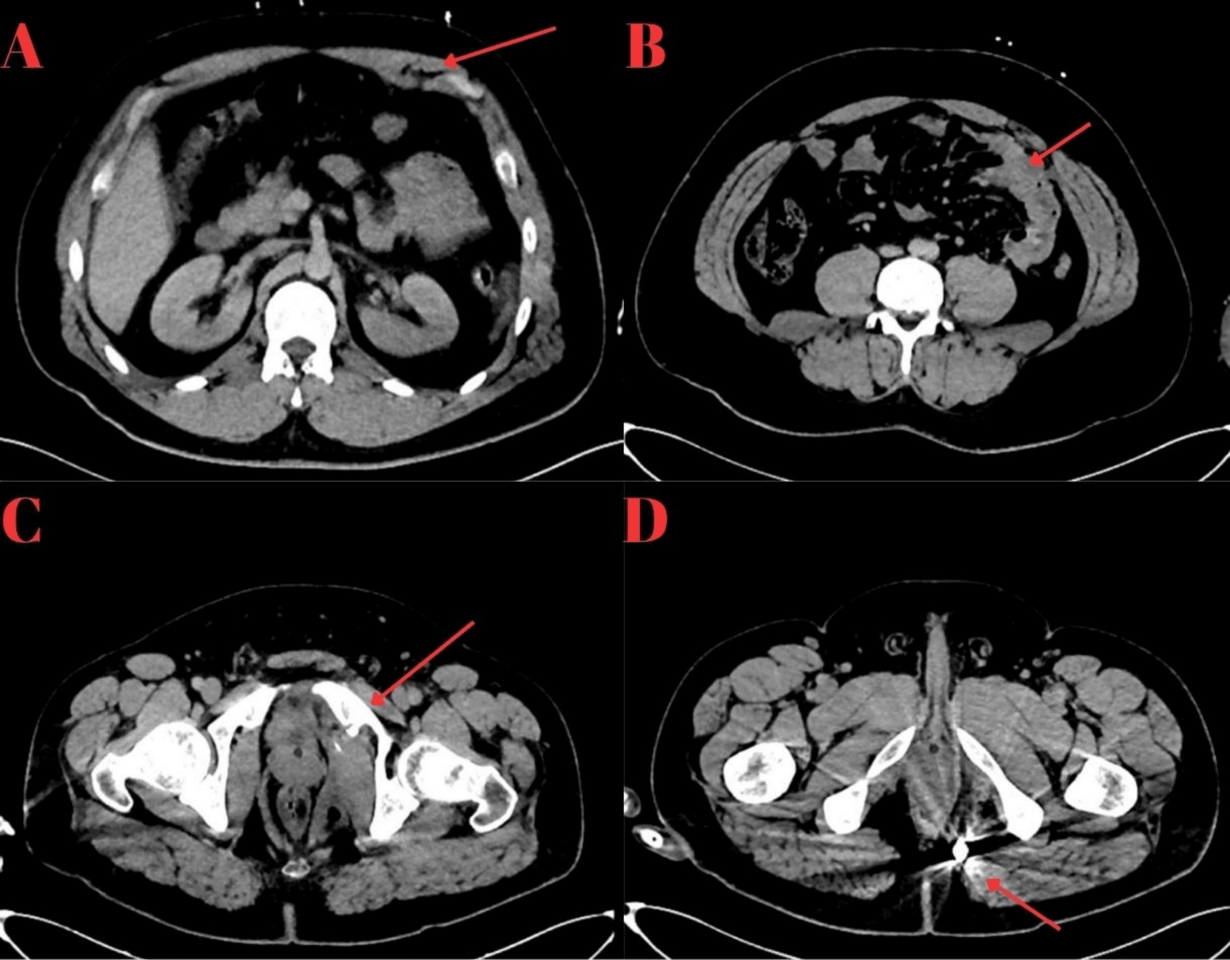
Contrast-enhanced CT scan images of the abdomen and pelvis (A) The arrow indicates free air in the anterior abdominal wall. (B) The arrow shows a suspected area of intraperitoneal hemorrhage adjacent to the bladder. (C) The arrow points to a fracture line in the left pubic ramus. (D) The arrow identifies a metallic foreign body lodged in the left gluteal region. CT: computed tomography
